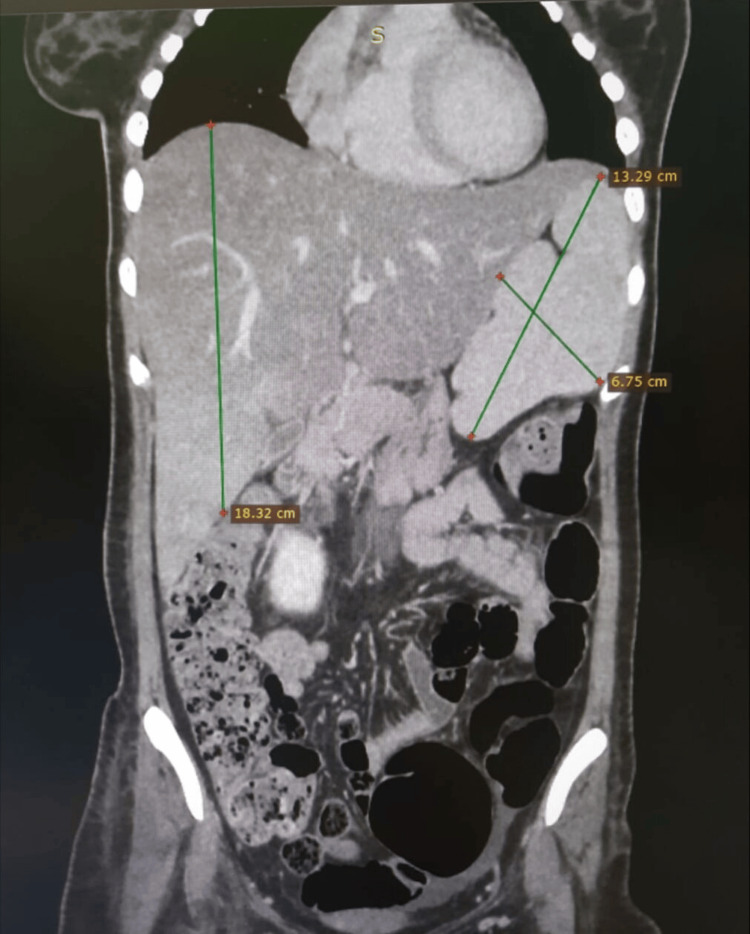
Contrast-enhanced CT of the abdomen showing moderate hepatomegaly (18.3 cm in size) and splenomegaly (13.29×6.75 cm in size) CT: computed tomography
